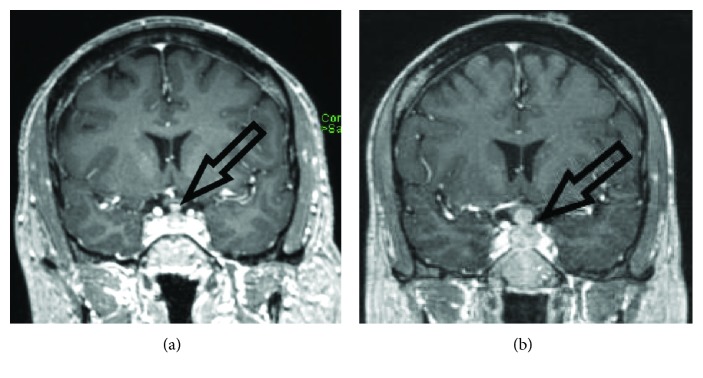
Brain magnetic resonance imaging demonstrating enlarging pituitary mass. (a) T1-weighted MRI demonstrating enlarged pituitary gland containing a hypovascular 10.5 × 7.5 mm mass lesion with thickening of the pituitary infundibulum without intracranial hemorrhage or extra-axial fluid collection. (b) Imaging one month later with T1-weighted MRI demonstrating a 22.0 × 12.0 mm bilobed mass with 8 mm suprasellar extension exerting mass effect on overlying optic chiasm.
